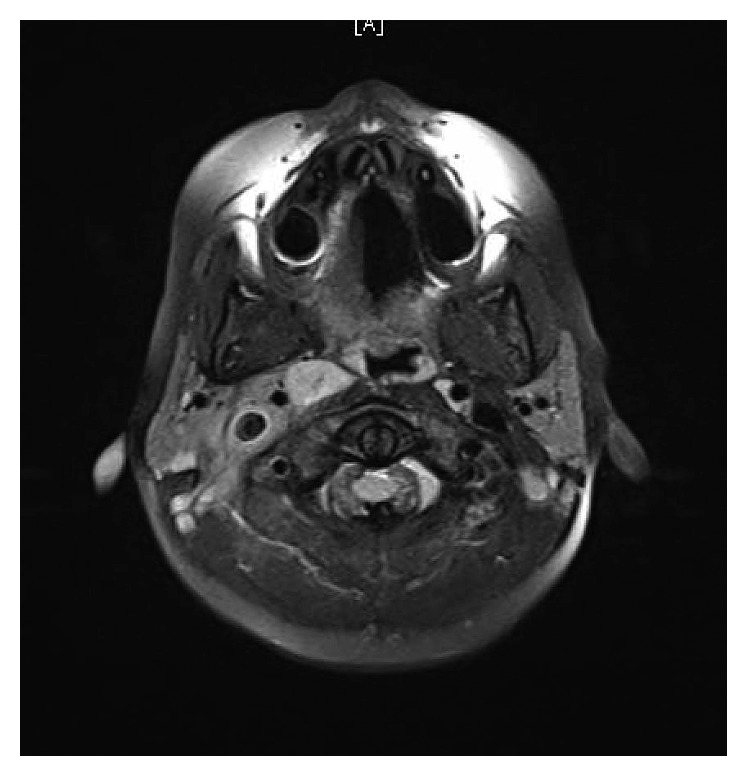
Horizontal section of the MRI of the brain.
